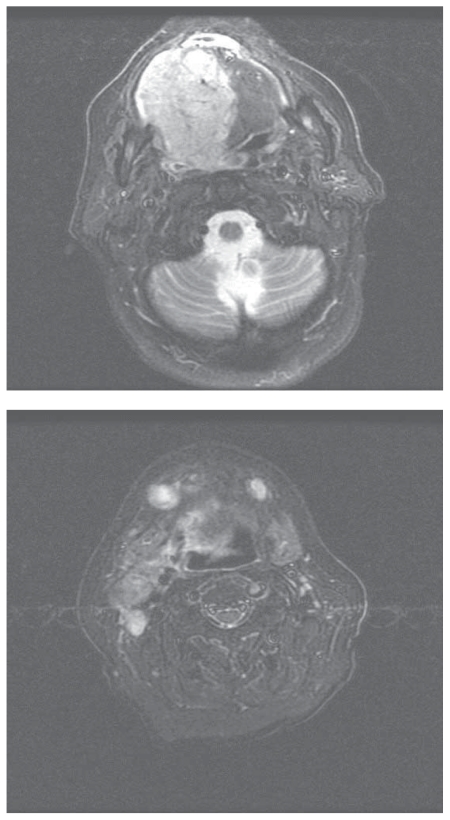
Pretreatment MRI. Axial fast spin-echo T2-weighted image with fat saturation at the level of the primary tumor and upper neck.
